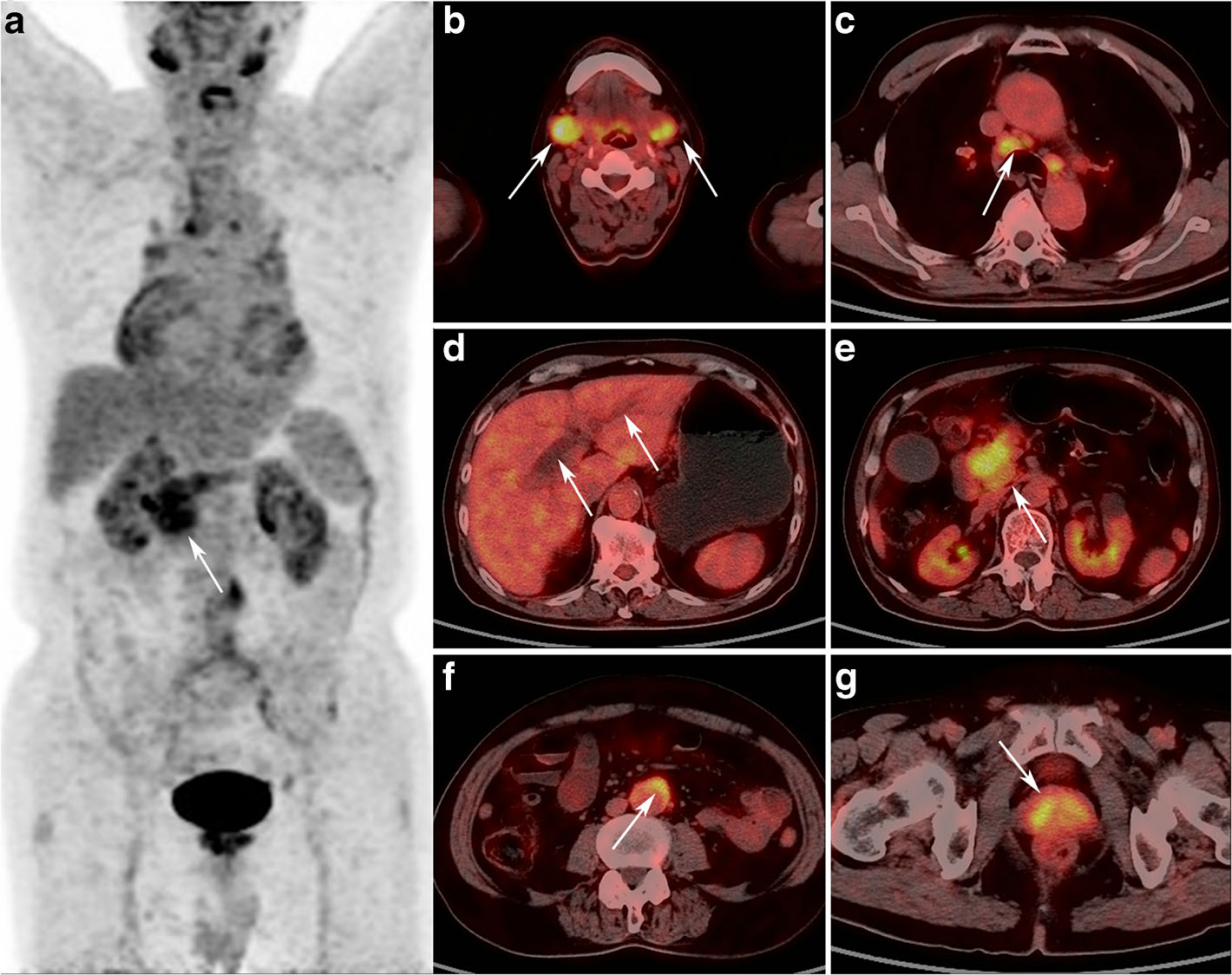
A 66-years old male patient with focal AIP in the pancreatic head. MIP PET (a) and PET/CT fusion (e) images shows localized enlargement of the pancreatic head with increased FDG uptake (arrow), with early and delayed SUVmax of 6.7 and 8.0, respectively. PET/CT fusion images shows (b) increased FDG uptake in bilateral submandibular gland, with a SUVmax of 7.9; (c), enlargement of mediastinal lymph node with increased FDG uptake (SUVmax, 5.7); (d), dilatation of bile duct; (f), retroperitoneal fibrosis around artery; (g) inverted “V” shaped high FDG uptake in the prostate
